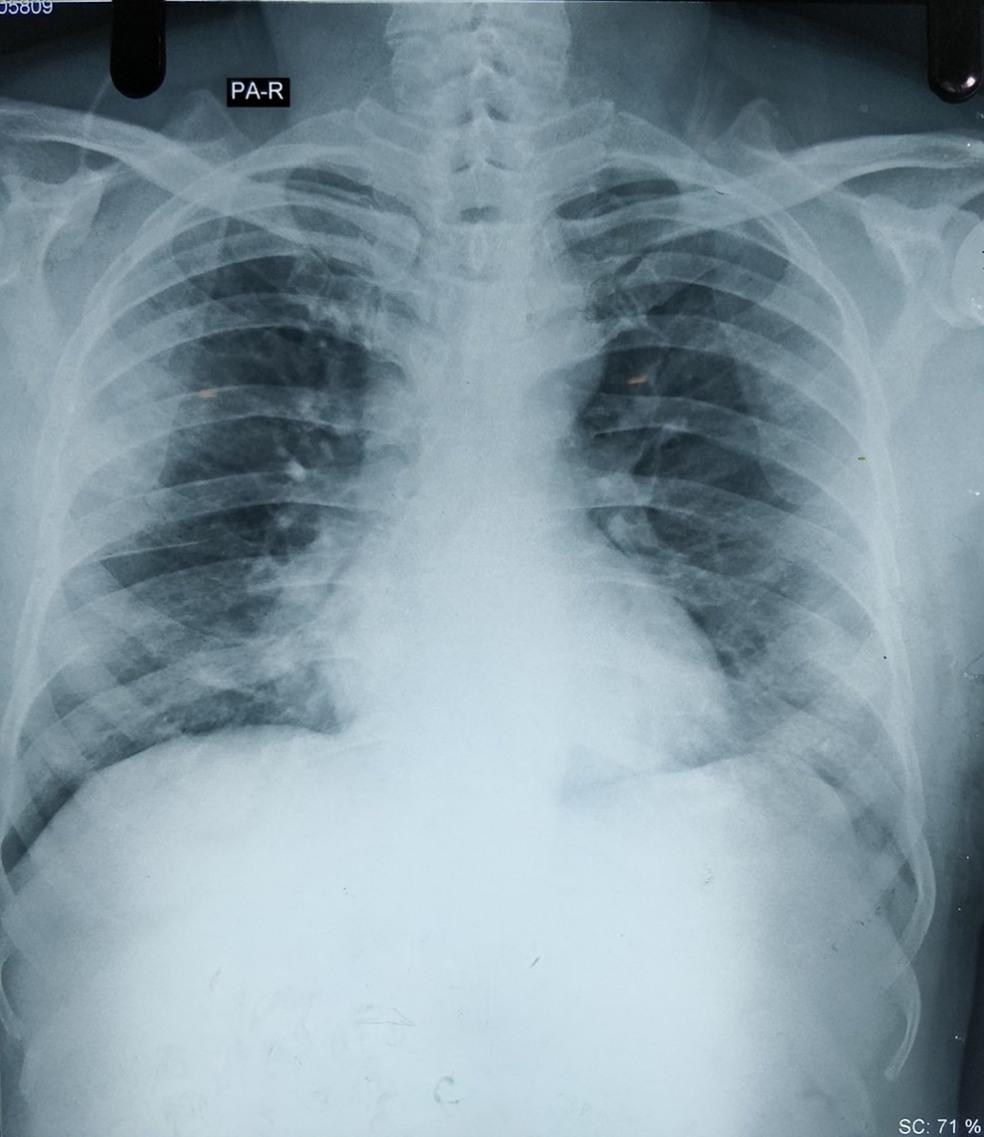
Chest X-ray demonstrating bilateral pneumonia.
